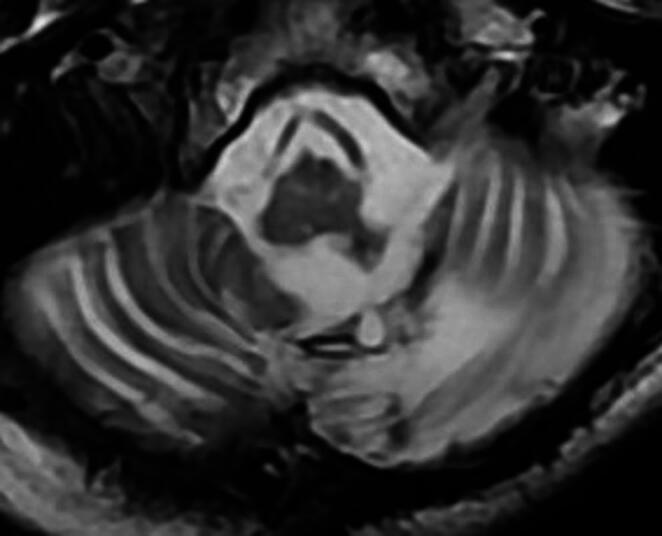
T2-weighted image showing high signal intensity in the left medullary and cerebellum
